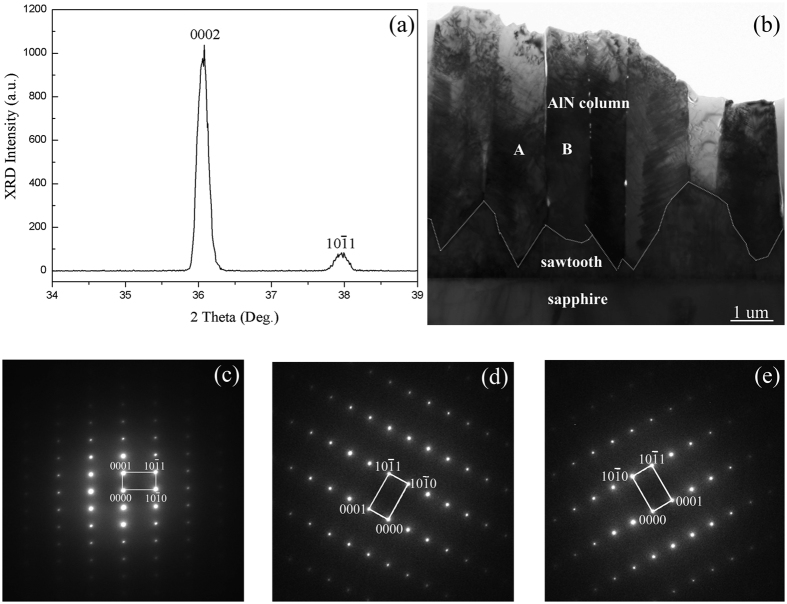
Characterization of AlN film grown on *c-plane* sapphire. (a) X-ray 2θ/ω scan of AlN thick film; (b) Cross-sectional TEM image of AlN thick film, which contains the sawtooth layer and the columnar layer; (c) SAED pattern taken from the sawtooth layer of (b); (d,e) are the SAED patterns taken from the neighboring column A and B in (b), respectively.
